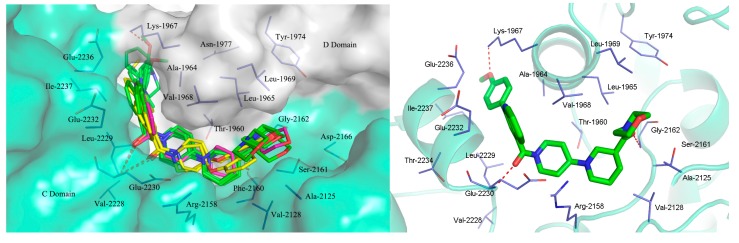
(Left) Overlay of the CT domain of hACC2 with the compounds of 7a, 7g, 7h, 7i (green), 12a, 12e (yellow) and CP-640186 (red), respectively. Red dashed lines denote the hydrogen bond. Molecular surface of the binding site is colored in white for the N domain and cyan for the C domain; (Right) Molecular docking model for compound 7a (green and stick) with the active site of hACC2, highlighting the hydrogen bonds (red dashed lines) coordination between the oxygen atoms in 7a and the amino acid residues Glu-2230, Gly-2162 and Lys-1967.
